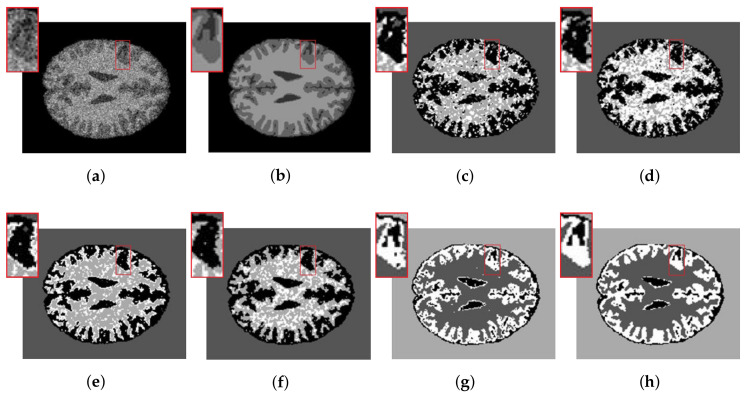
32% Rician-noised synthetic MR images: (a) original; (b) ground-truth image and after segmentation into four regions with (c) SFCM; (d) SSCM; (e) NLSFCM; (f) NLSSCM; (g) $FCE_{\_KL}$; (h) $FCE_{\_sKL}$.
